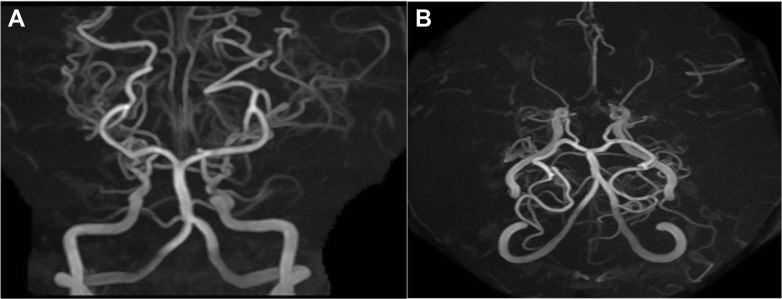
Head Magnetic Resonance Imaging (A and B) Head magnetic resonance imaging revealed occlusion of both terminal internal carotid arteries with associated abnormal collateral vascular networks, consistent with the diagnosis of moyamoya disease.
